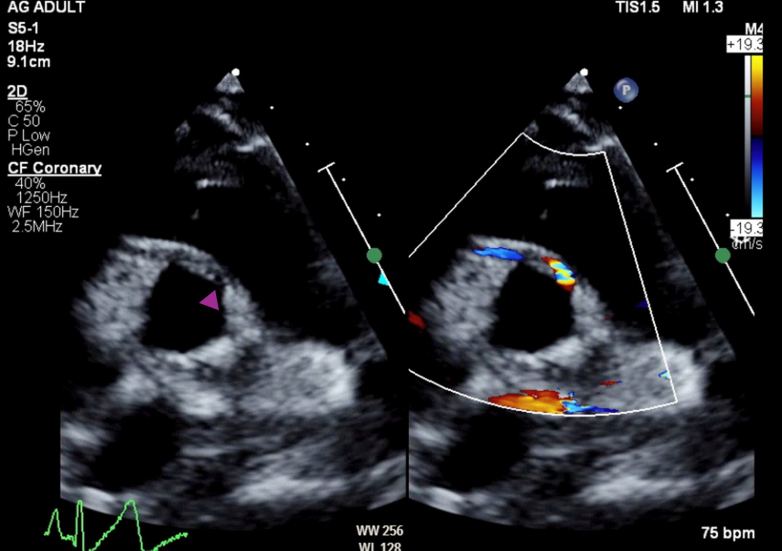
Initial Transthoracic Echocardiogram Transthoracic echocardiography, parasternal short-axis view, simultaneous 2-dimensional and color imaging, anomalous origin of the right coronary artery from the left-facing coronary sinus (purple arrowhead). The aortic origin of the left main coronary artery left main coronary artery was unable to be clearly seen.
